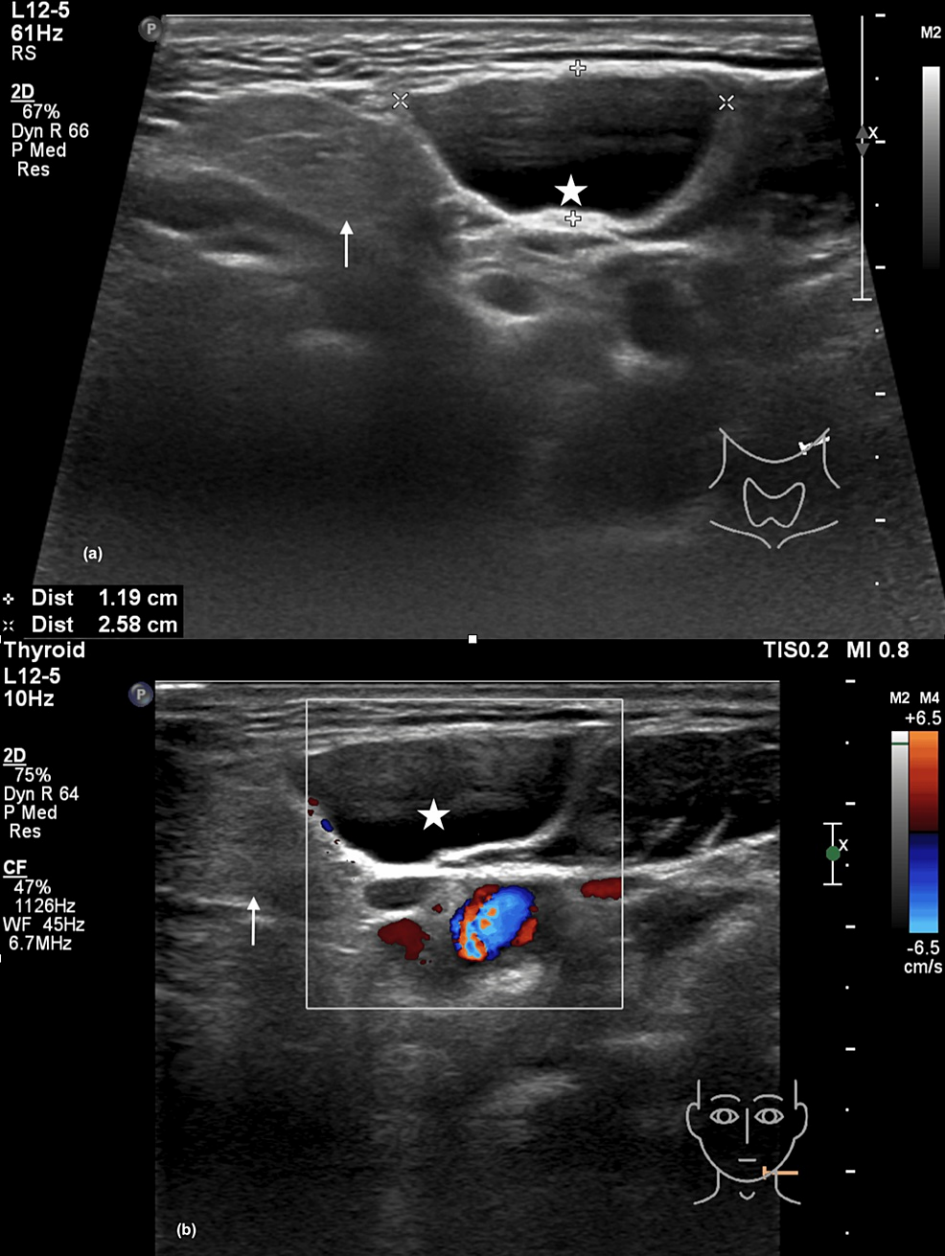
Ultrasonography of the neck (a) An anechoic mass (star) with well-defined margin and thin walls, located anteromedial to the left sternocleidomastoid muscle, and a normal submandibular gland (arrow); (b) the mass demonstrated no internal vascularity on color doppler.
